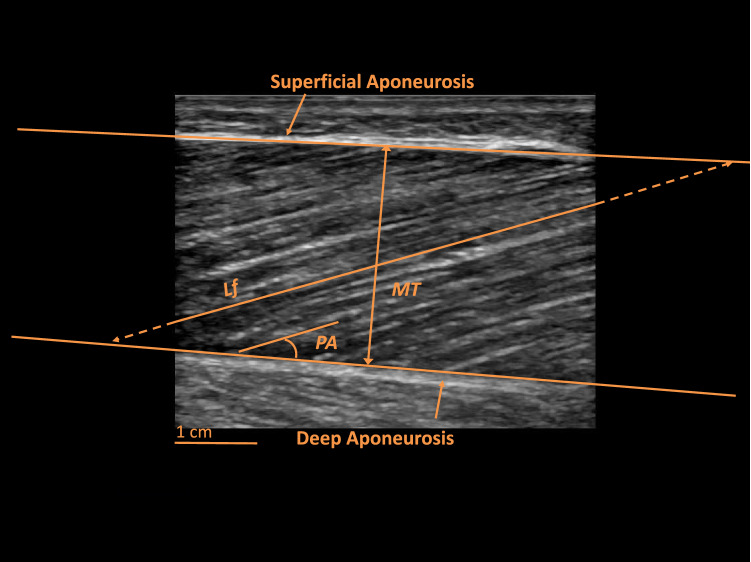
Longitudinal B-mode ultrasound image of a human vastus lateralis muscle captured at 50% of the total femur length. Highlighted are the deep and superficial tendon aponeuroses and the most common features of muscle architecture: *MT* muscle thickness, *Lf* fascicle length, *PA* pennation angle. Image was obtained using a 4.7 cm linear transducer
